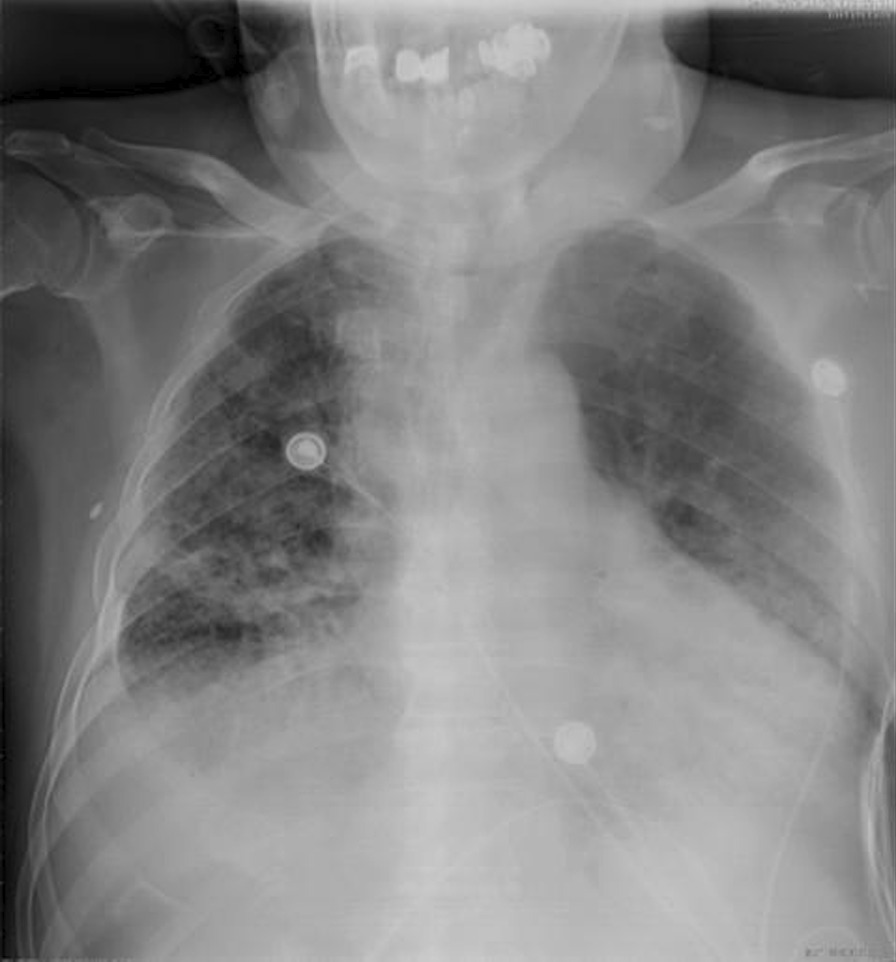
Bedside chest X-ray. Multiple lungs with patchy high-density shadows
